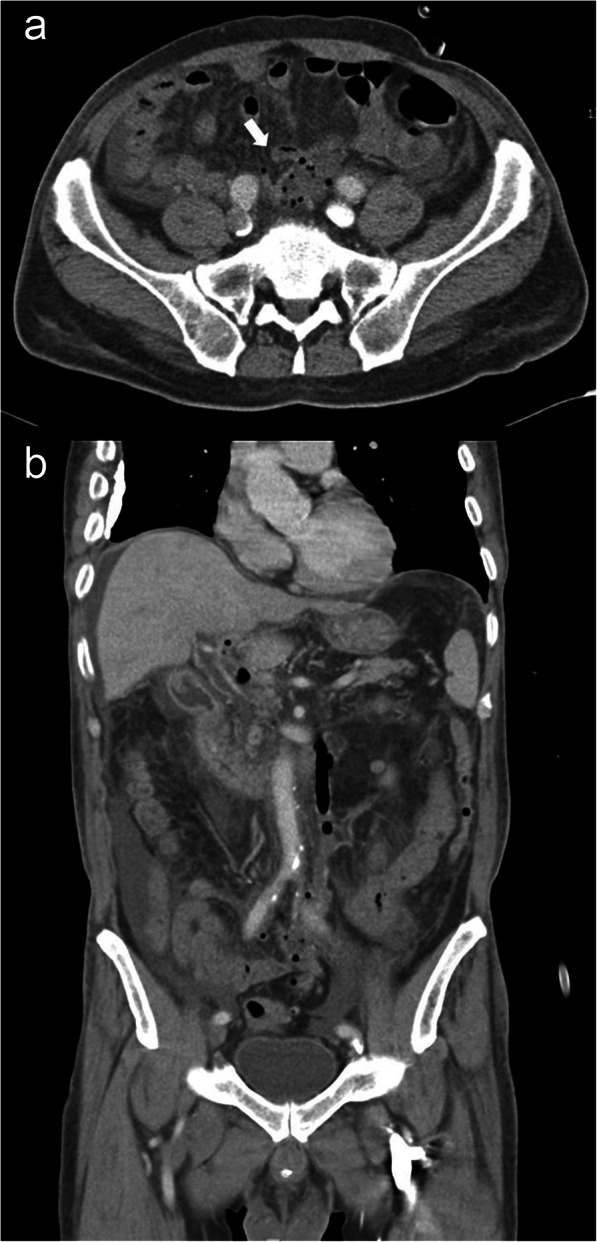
Follow-up CT showed a 4.1 × 4.0 cm RA (a arrow) and deteriorated thrombophlebitis of the portal venous system (b)
